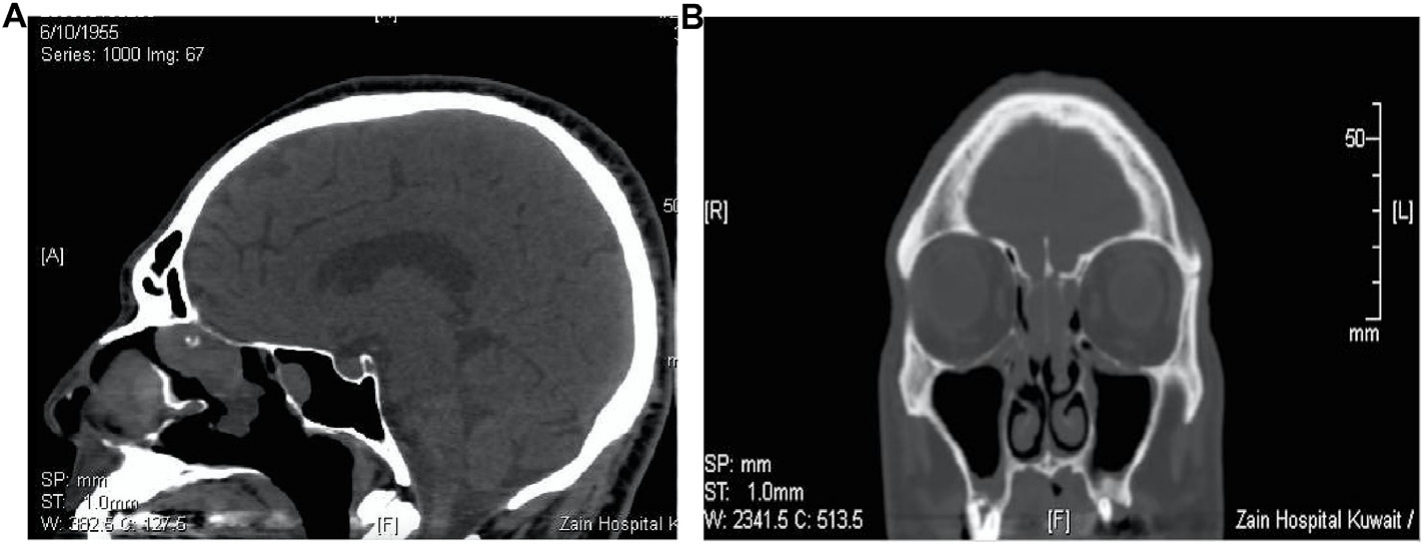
A & B: Sagittal (A) and Coronal (B) soft tissue window CT sinus showing bilateral polypoidal soft tissue lesion present at the roof of nasal cavity with intact overlying bony floor of anterior cranial fossa, no intracranial extension on CT basis.
